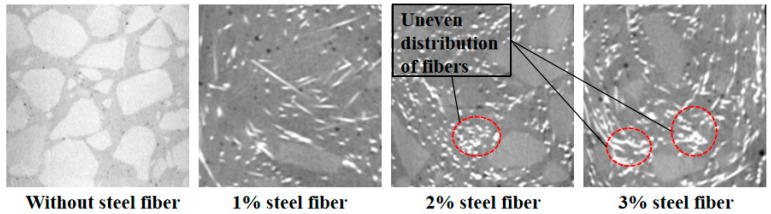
CT images of UHPC-CA with different steel fiber contents.
